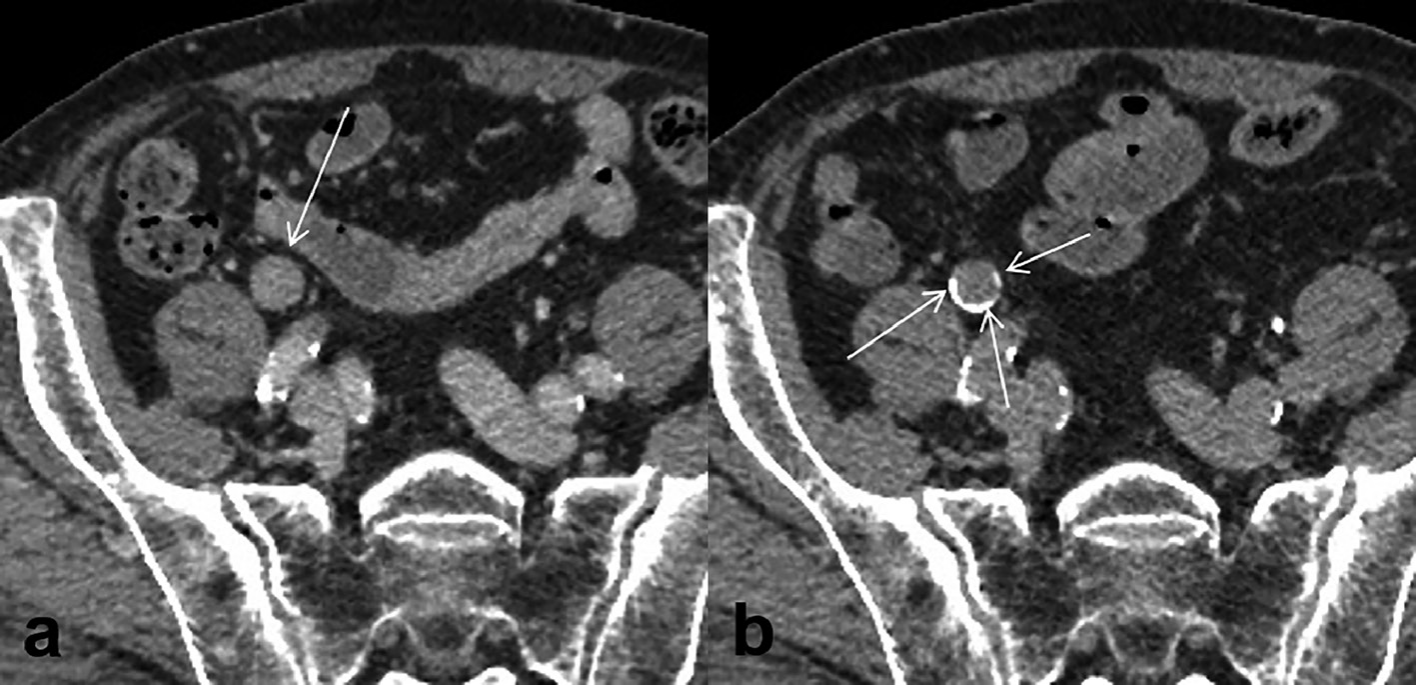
CT urography [(a) nephrographic phase; (b) excretory phase] A homogeneous mass (a: arrow) is visible in the right middle ureter during the nephrographic phase, and contrast medium retention (b: arrow) is seen around the mass during the excretory phase. Therefore, the mass can be interpreted as a papillary tumour growing within the ureteral lumen.
